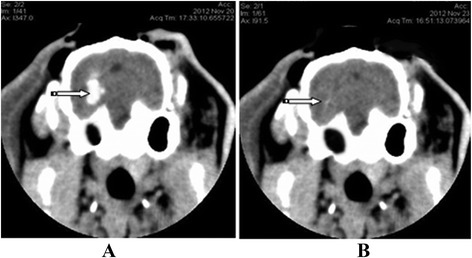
Brain CT showing ICH before and after minimally invasive surgery. A: The hyper-dense area (the arrow) in the brain CT showing successful preparation of the ICH model. B: The ICH was removed after minimally invasive surgery.
